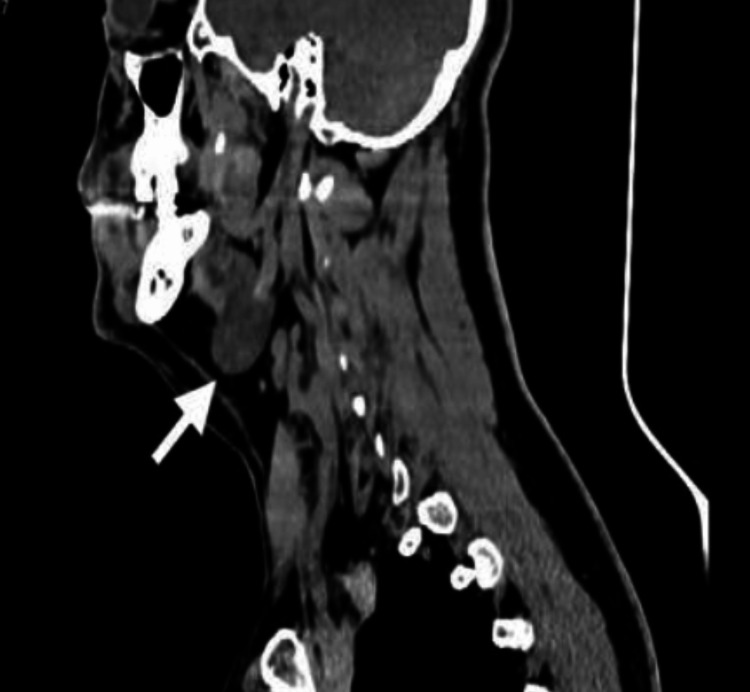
Sagittal CT scan showing a well-defined hypodense lesion with regular borders in the submandibular region.
